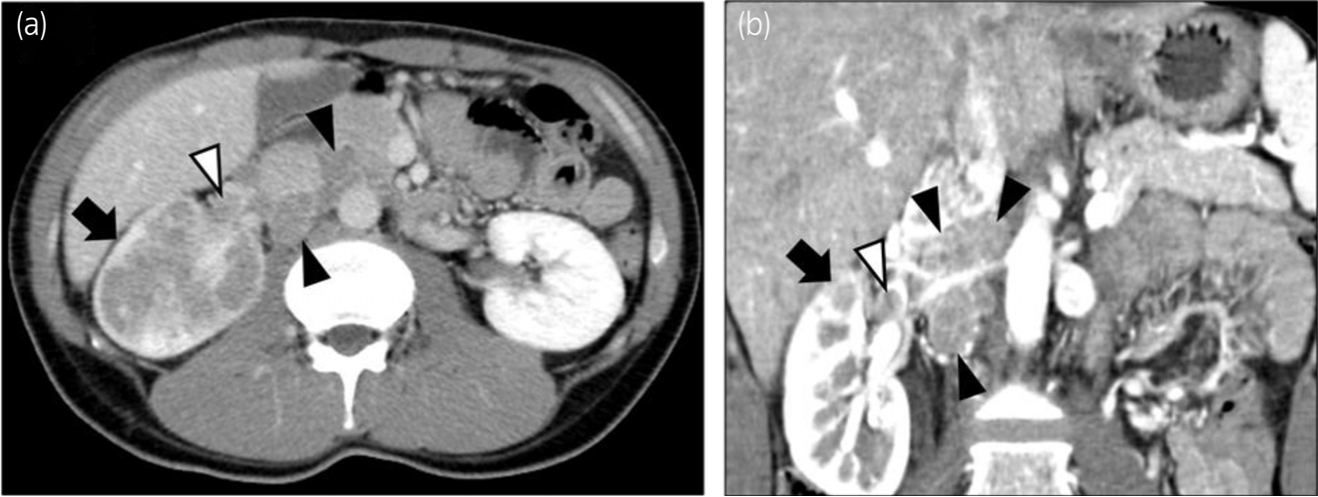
Abdominal contrast CT shows a right renal tumor (Black arrow) of 66 mm in size with thrombus (White arrow heads) in right renal vein and multiple enlarged LNs (Black arrow heads). (a) Axial image, (b) Coronal image.
